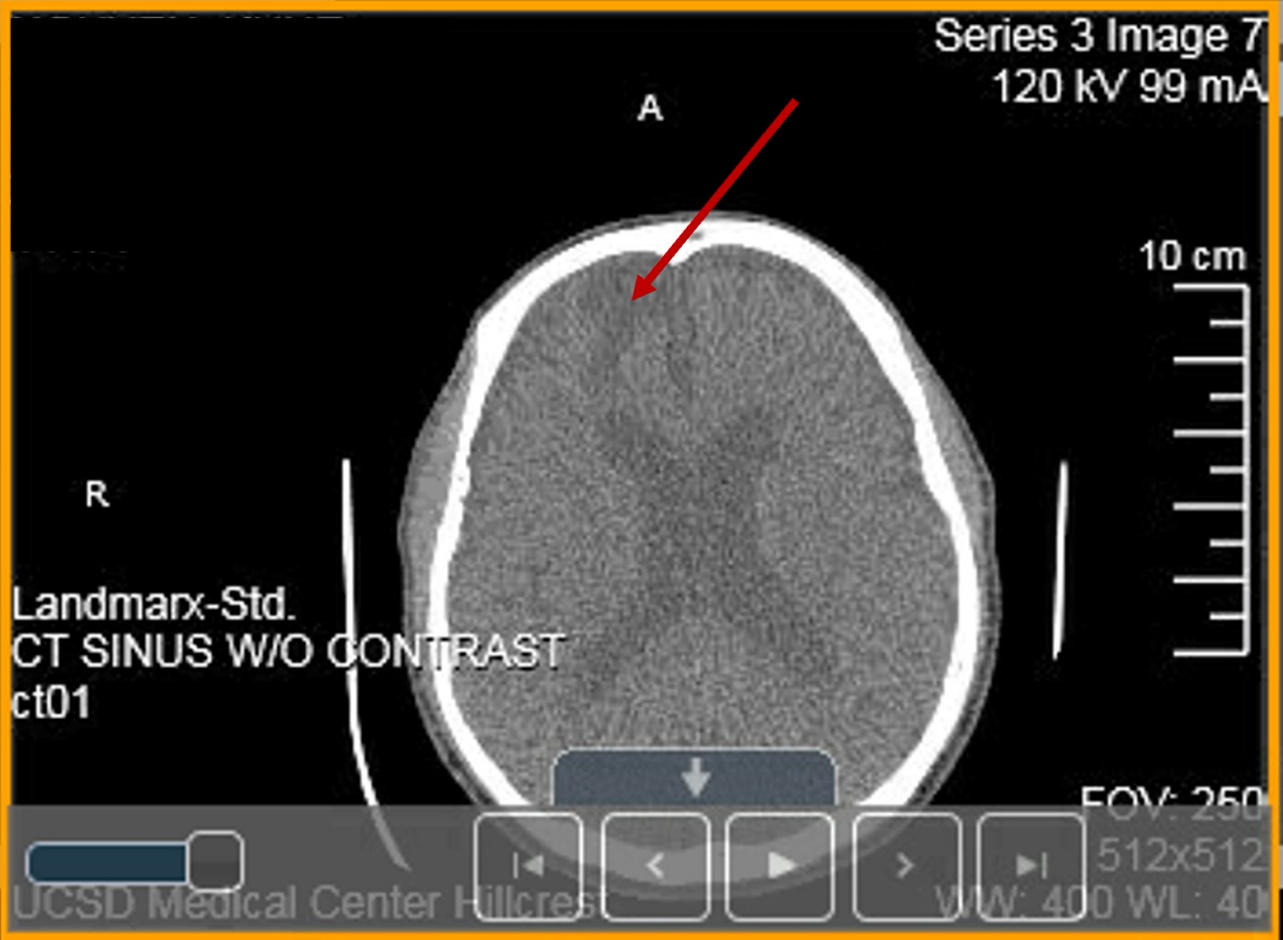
Non-contrast cerebral computed tomography (CT) scans of the paranasal sinuses performed with 0.625 mm axial slices, reformatted in the coronal and sagittal planes. Limited visualized portions of the brain demonstrate encephalomalacia/gliosis involving the anterior right frontal lobe suggesting sequel of prior trauma (dark area at the top-left corner of this image, red arrow).
